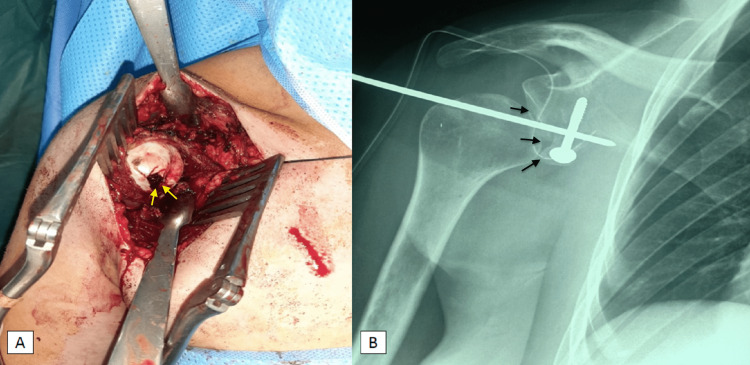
(A) Intraoperative view showing the bone defect on the right humeral head (yellow arrows) with loss of contact between the humeral head and the glenoid cavity. (B) Frontal postoperative X-ray showing reduced glenohumeral joint (black arrows) fixed with a Kirschner wire and one screw in accordance with the Latarjet procedure.
